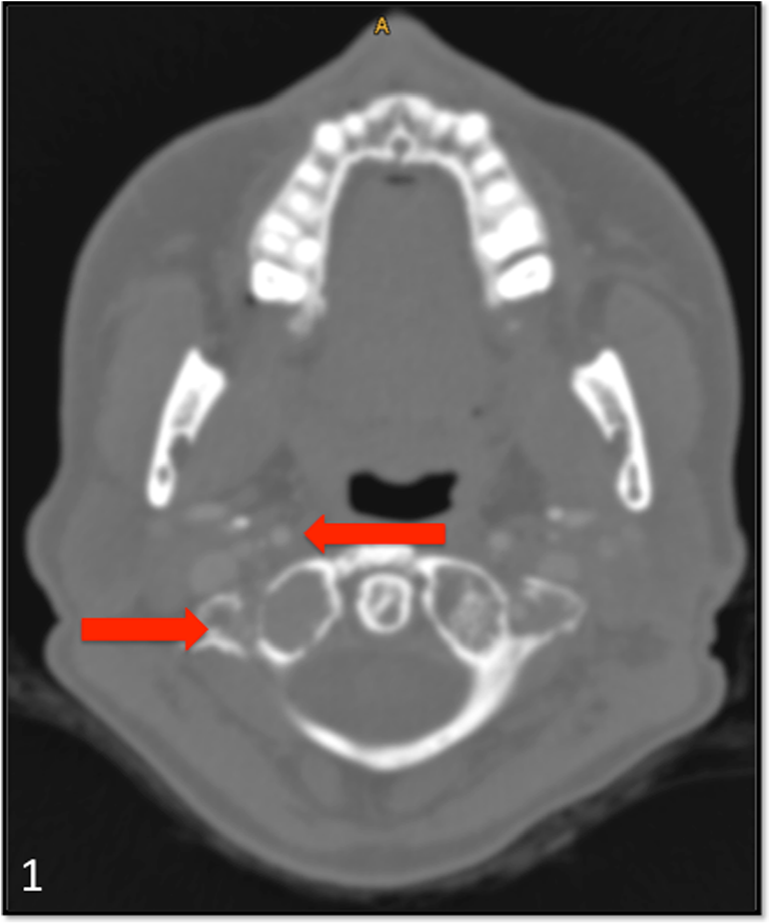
CT angiogram of an osteolytic lesion in the right lateral mass of the atlas in a patient with multiple myeloma. *Arrows* show the internal carotid and vertebral arteries
